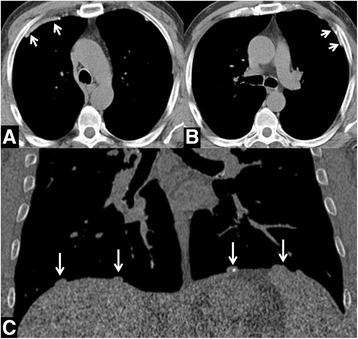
a-c Pleural plaques. a-b - Transverse CT image of the chest shows smooth solid thickening of pleural surface with clear-cut edge. c – Coronal reconstruction of the chest shows smooth solid thickening of pleural surface above the diaphragm, with scant calcification
